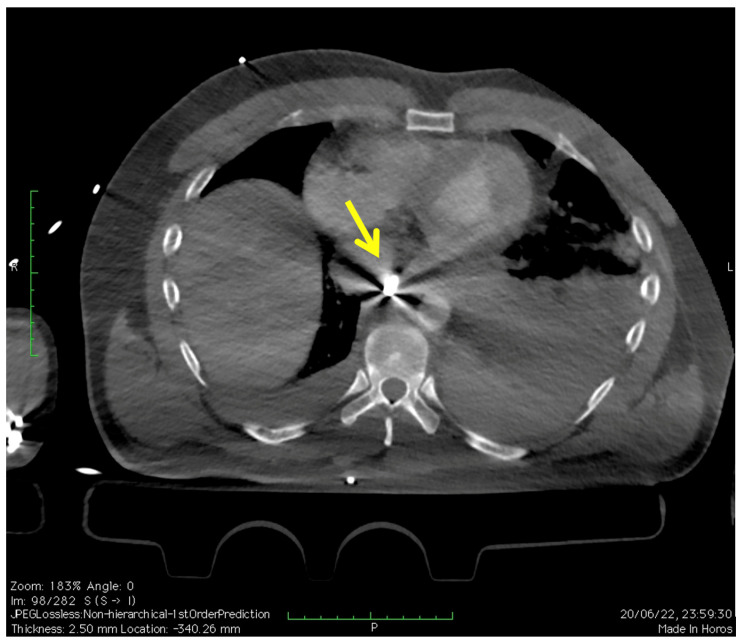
CTA of the chest revealing penetrating thoracic aorta injury (transversal view). Yellow arrow shows the bullet.
